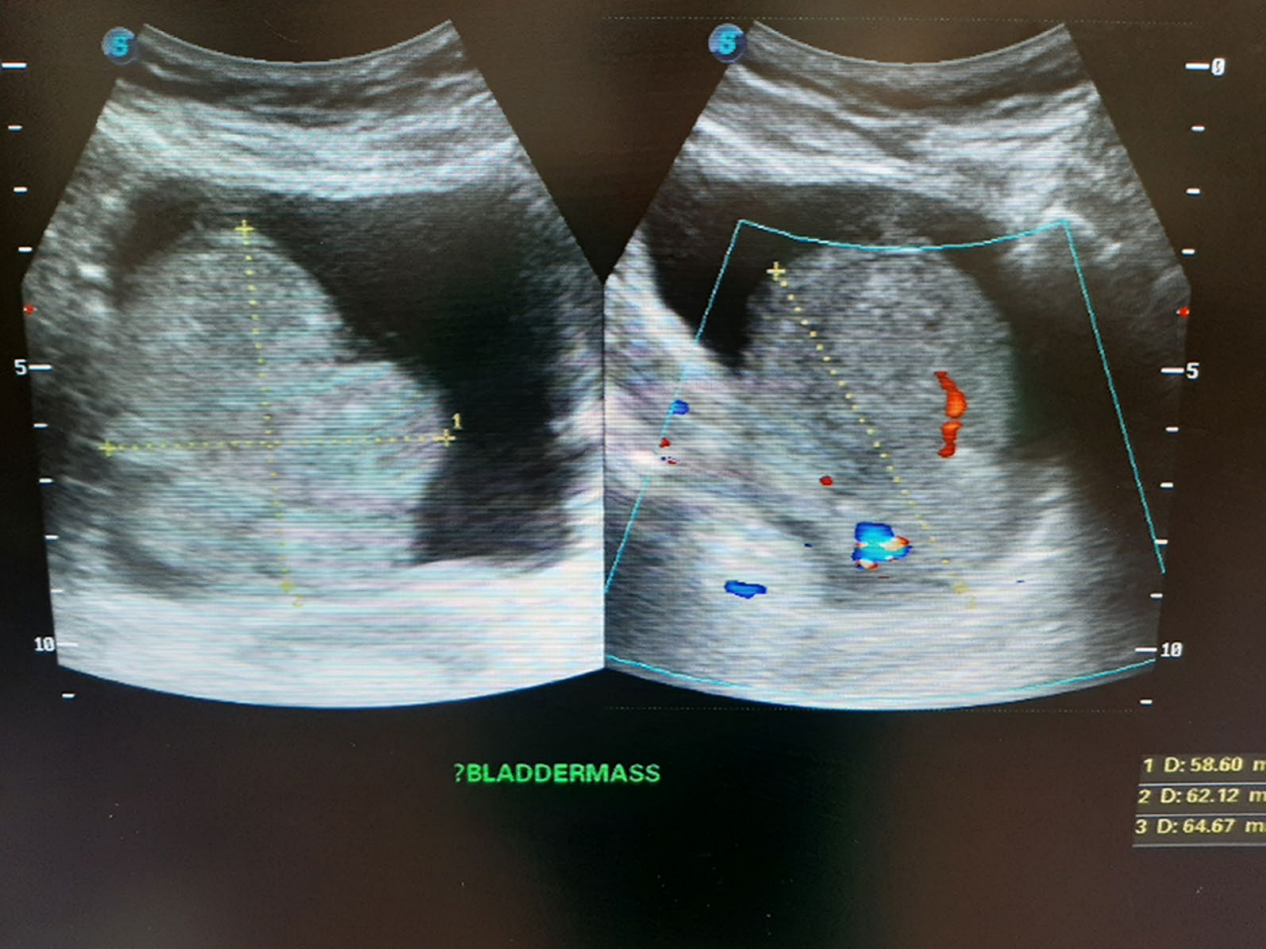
Ultrasound with bladder mass
